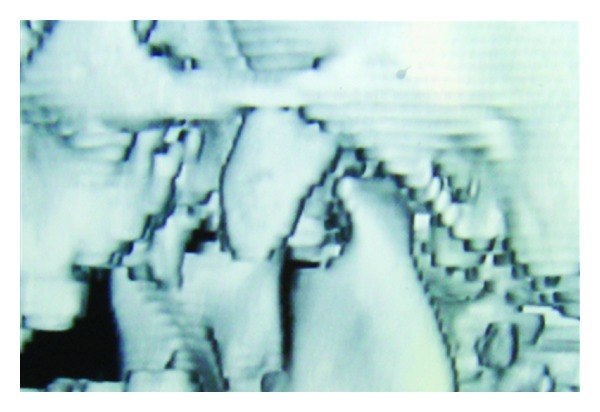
Computed tomography-lateral view.
